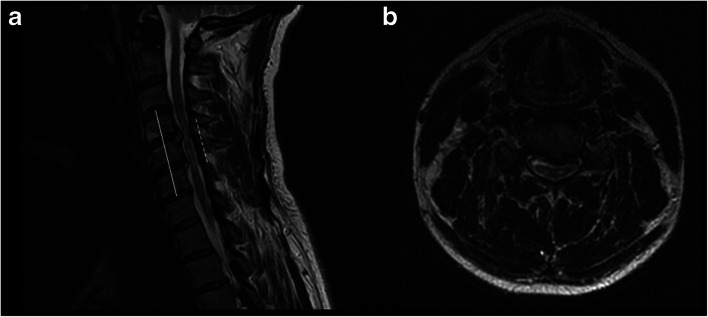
MRI of a patient with central canal stenosis and myelopathy. Sagittal T2wi MR shows severe central canal stenosis extending from C4 to C6 levels with reduction of subarachnoid spaces. The central cord hyperintensity is documented to the maximum spinal cord compression level extending from C4 to C5 (a). Gray matter intramedullary T2 hyperintensity is well shown on axial T2 images (b)
